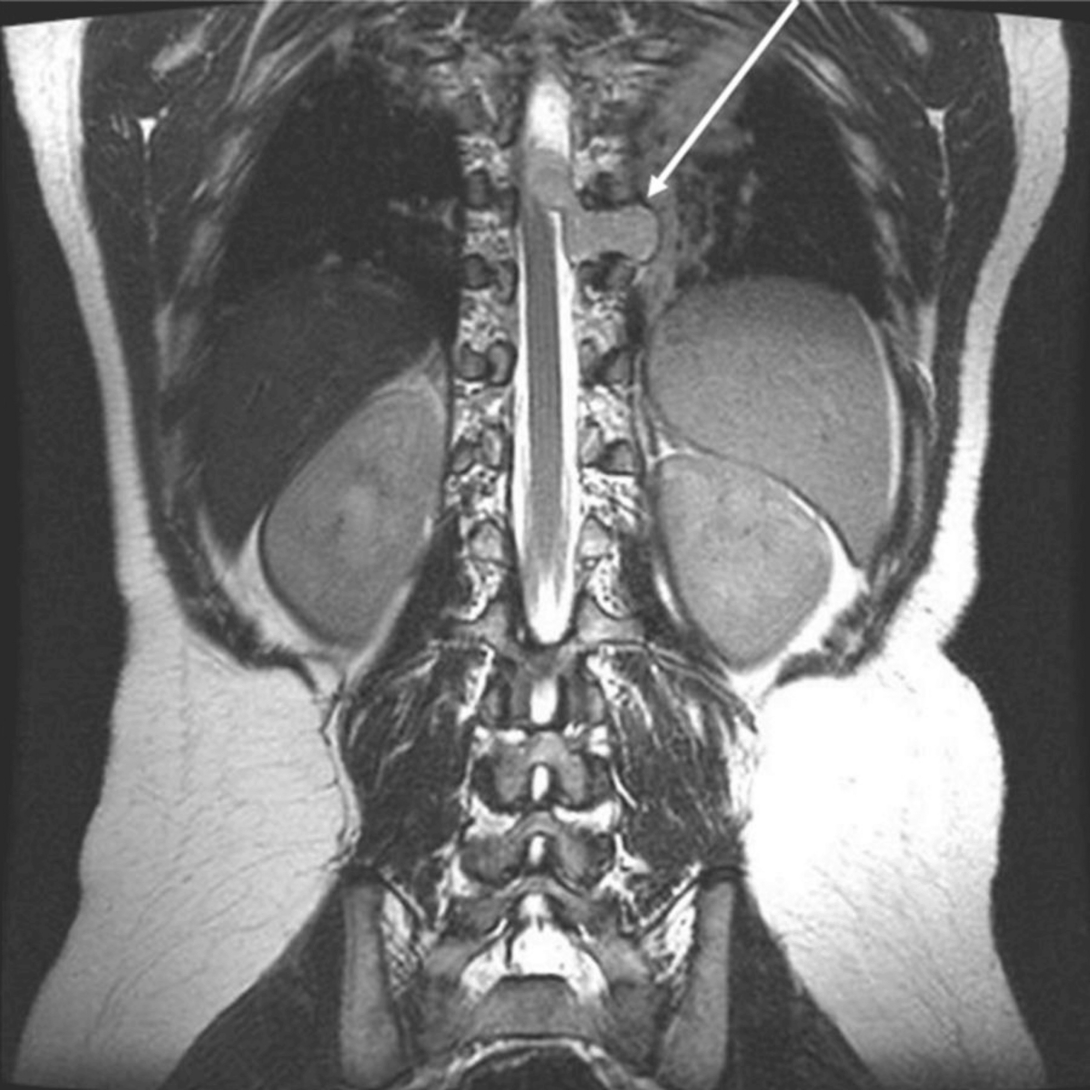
Spine MRI (T2 coronal view) showing extension of the tumor within the spinal canal with significant spinal cord compression (arrow).
